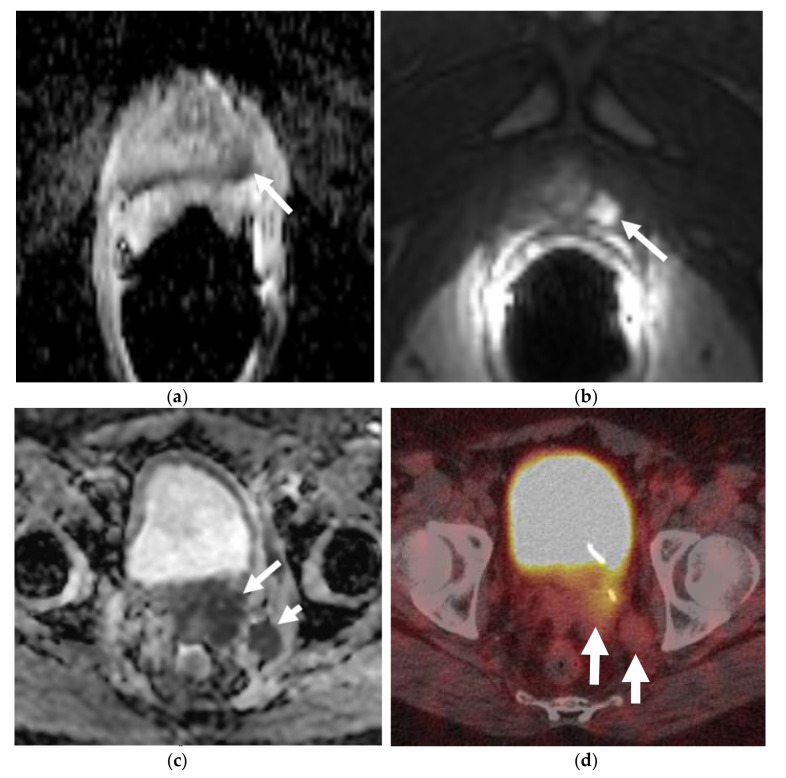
A 56-year-old male with prior hormonal therapy for 2 years and external beam radiation therapy for the biopsy-proven diagnosis of prostatic adenocarcinoma in 2008. Follow-up MRI pelvis performed for recurrent elevated serum PSA 6 years after shows a left posterolateral peripheral zone prostatic nodule with restricted diffusion on ADC map (a) and avid early enhancement on the dynamic postcontrast T1W images (b), suspicious for recurrent prostatic neoplasm (arrow). This was biopsy proven to be prostatic adenocarcinoma. Further follow-up MRIs after 12 years showed rapidly enlarging left prostatic neoplasm (long arrow) with regional left internal iliac lymphadenopathy (short arrow) on ADC image (c) along with metastatic left common iliac and retroperitoneal lymphadenopathy. This was biopsy proven to be metastatic prostatic neuroendocrine carcinoma. Prostatic mass showed heterogeneous hypermetabolism on the axial color fused image of the Ga-68 DOTATATE PET-CT (d). The nodal metastases (arrow) were without suspicious hypermetabolism due to poorly differentiated neuroendocrine neoplasm/carcinoma component.
